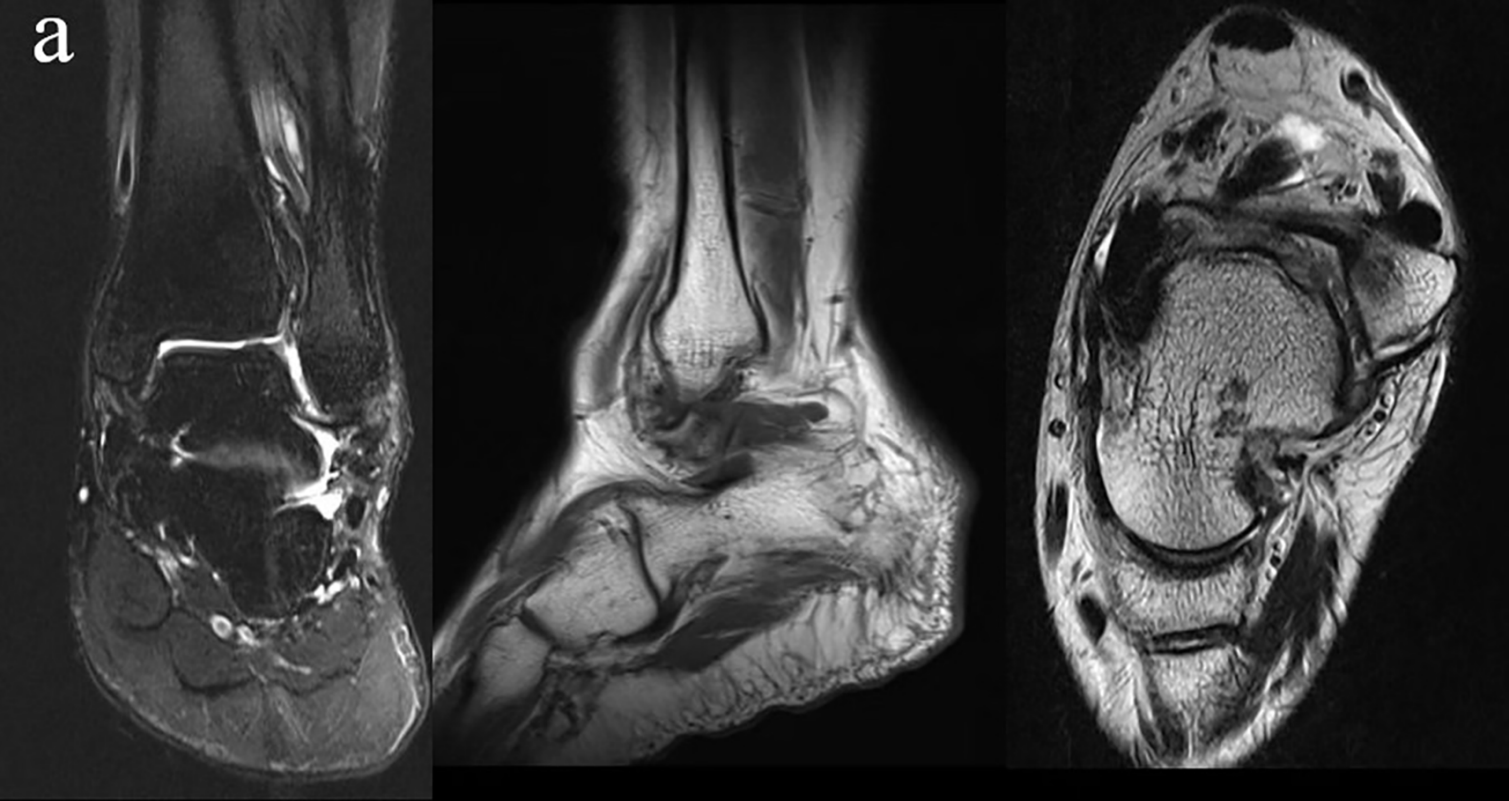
Preoperative MRI reveals an increased signal in the ATFL pathway, which is indicative of an ATFL injury. Additionally, effusion in the ankle joint and swelling of the surrounding soft tissues are observed.
